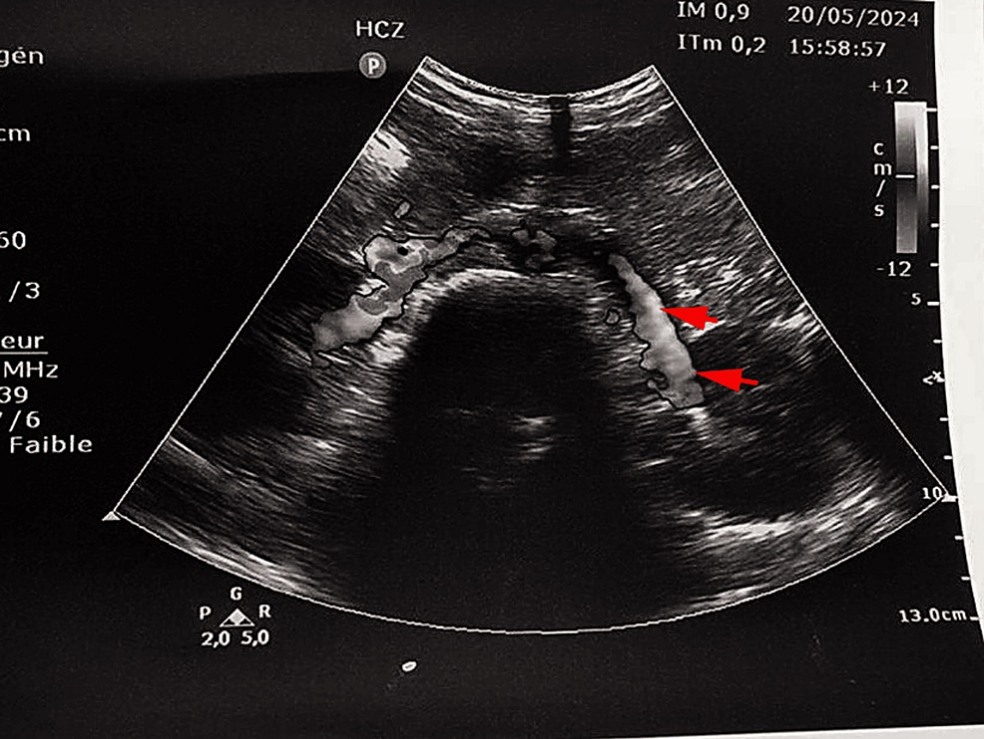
Ultrasound of the abdomen An abdominal ultrasound revealed ectasia of the left renal vein (red arrows).
